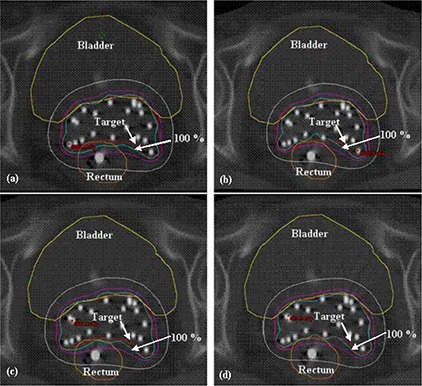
Isodose distribution on the same CT slice for (a) GO; (b) GO_IsoR; (c) VO; (d) VO_IsoR.
